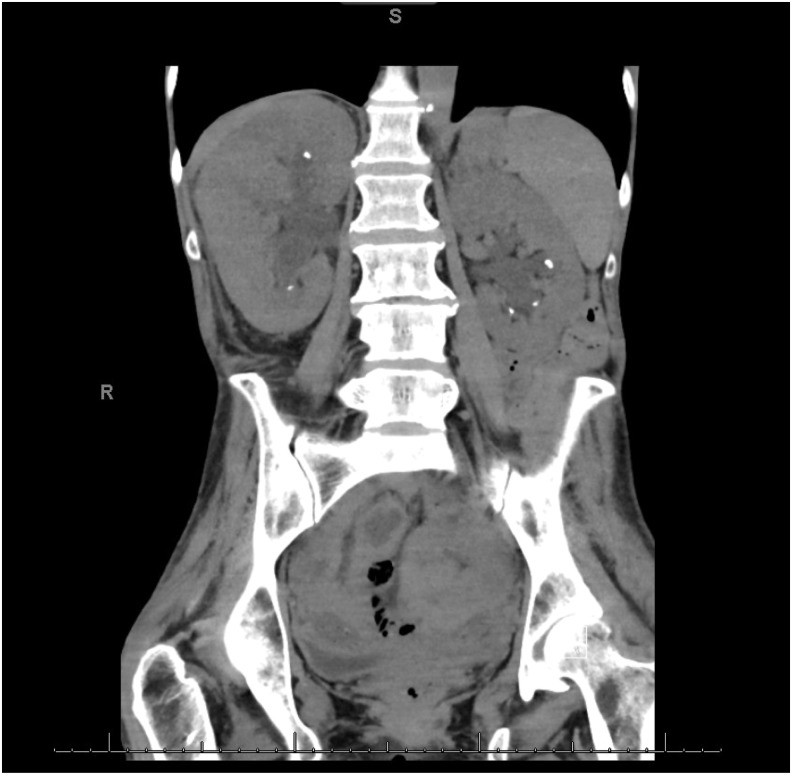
CT of abdomen and pelvis. Multiple bilateral renal stones measuring between 1 and 4 mm. Bilateral pelvocaliectasis. No discrete renal lesions although renal fungal ball cannot be excluded.
